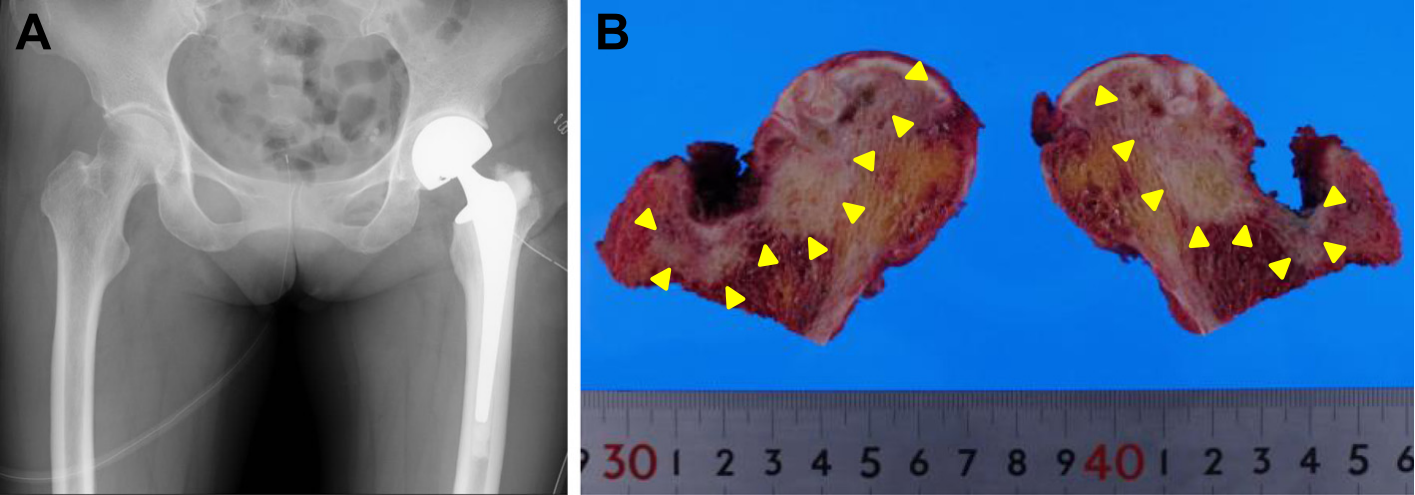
a X-ray, showing the left hip joint after BHA. b Photograph of the vertically divided left femoral head after surgery. DTC was observed in the subchondral region of femoral head (*arrowhead*). There was no cartilage discontinuity
